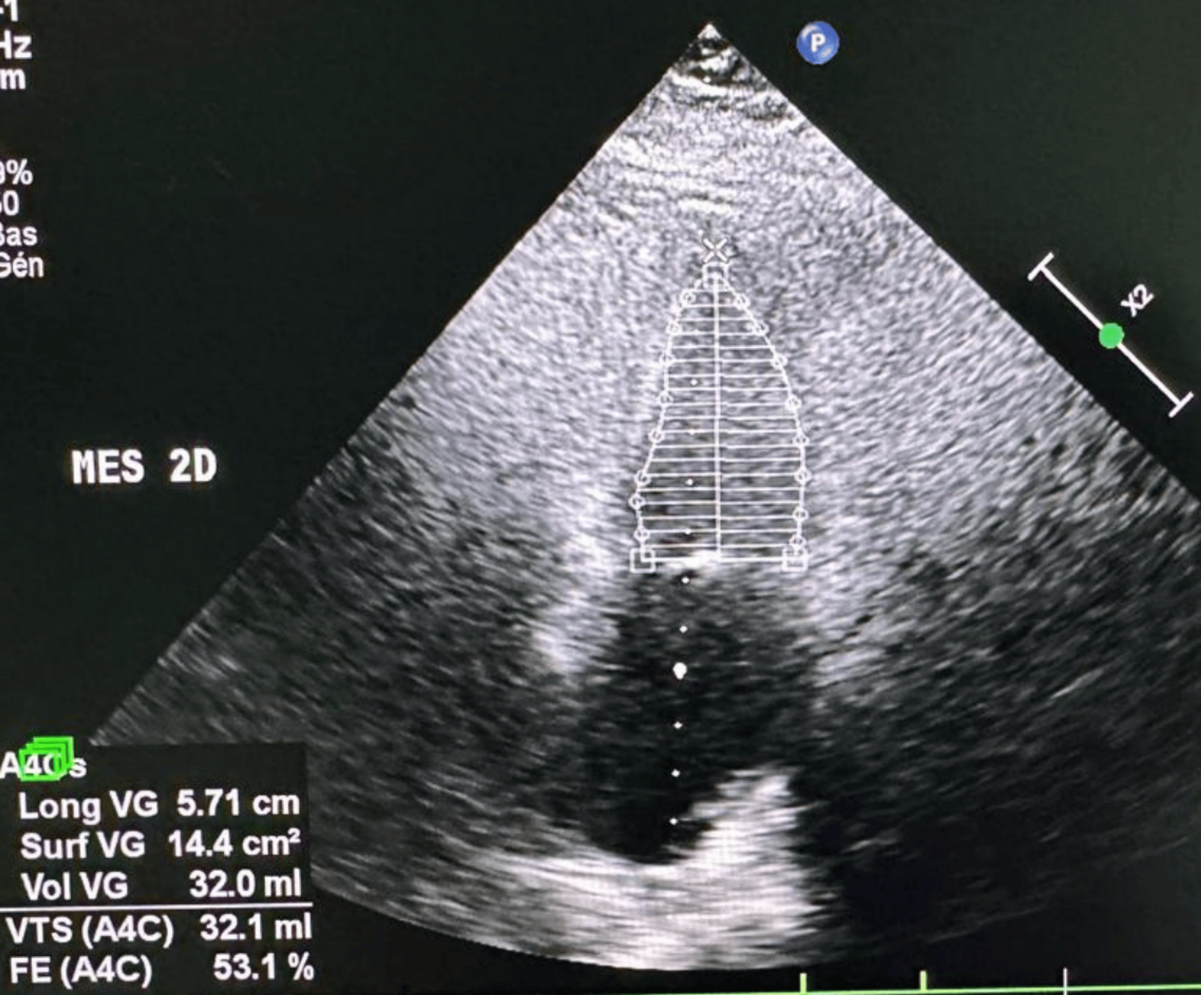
Transthoracic echocardiography. Apical two-chamber view demonstrating normal biventricular systolic function with a preserved left ventricular ejection fraction.
